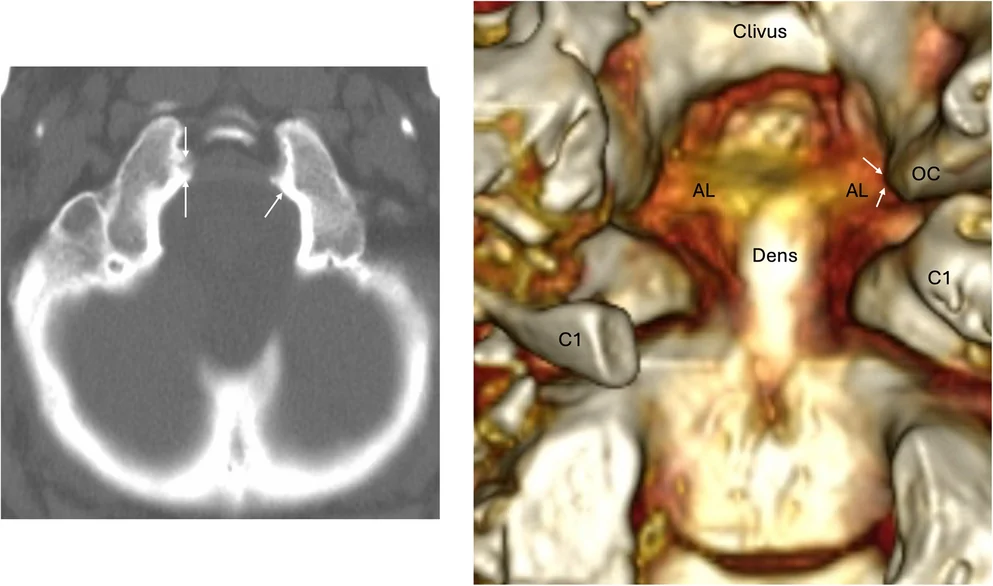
Two examples of imaging of the alar ligament tubercles. Left: Axial CT through the occipital condyles and noting left and right alar ligament tubercles (arrows). Right: 3D reconstruction (posterior view) of the cranial cervical junction and noting the left and right alar ligaments (AL) attaching onto the occipital condyles (OC). Note the alar ligament tubercle on the right side (arrows). C1 = atlas
